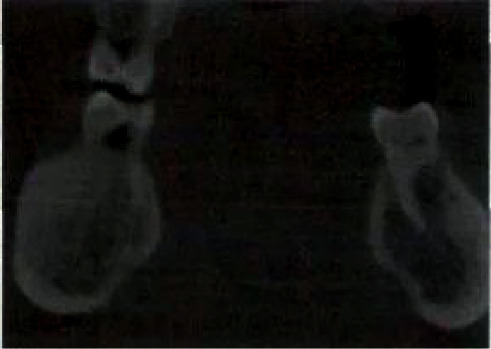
CBCT image of the lesion by DL segmentation.
